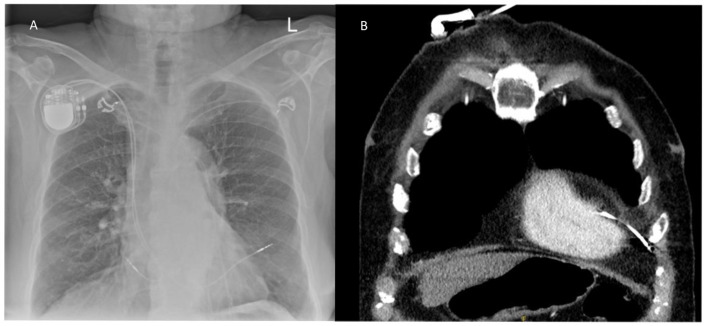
(A) Shows chest X-Ray with a right ventricular lead perforation, (B) Shows a computer tomography with right ventricular lead perforation.
